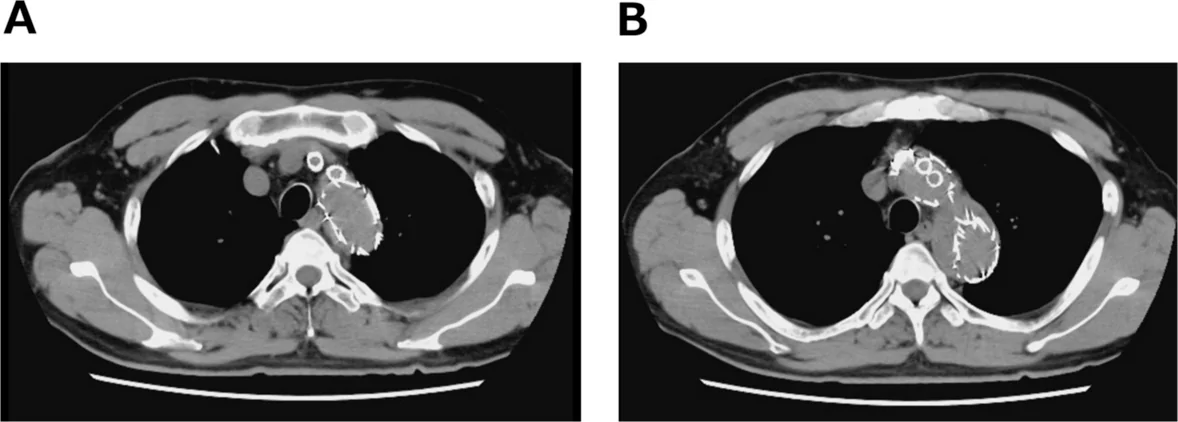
Axial plain CT images obtained at the 6-month follow-up (A) The supra-aortic branches remain patent. B The aortic aneurysm diameter measures 42 mm, representing no significant interval change. CT: computed tomography
